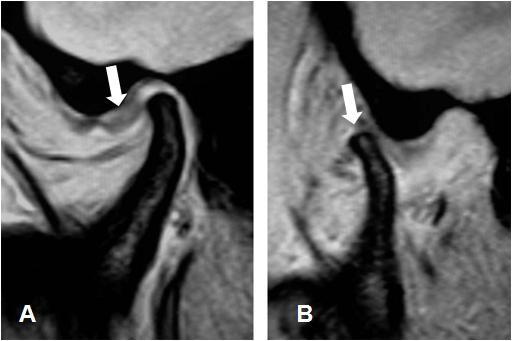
Magnetic Resonance Imaging (MRI). The MR scan of the TMJ with the mouth closed and open. For details see text.
